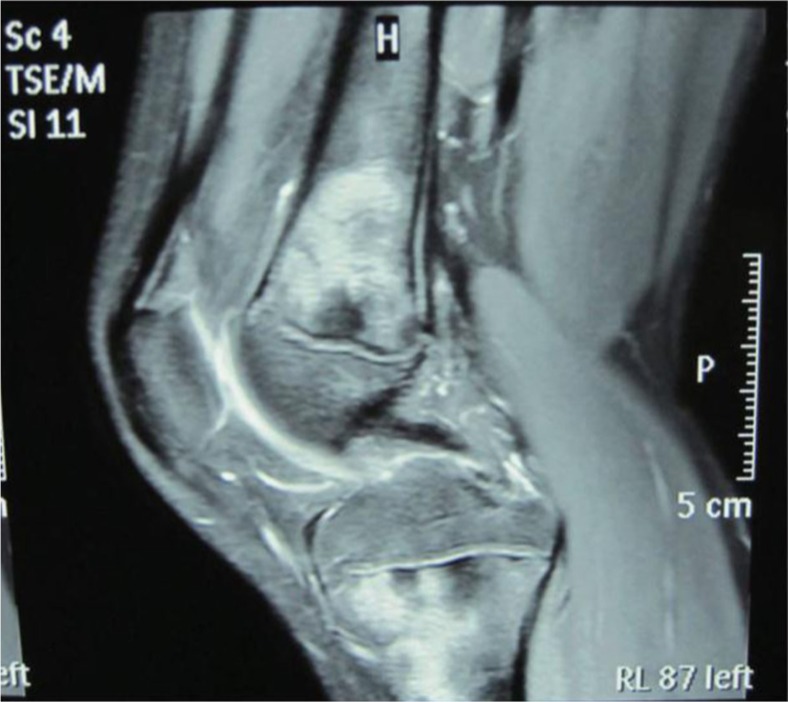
MRI of the left knee showing marked flakes and nodular long signals on T2-weighted MRI in the distal femur, proximal tibia, and even the osteoephysis.
